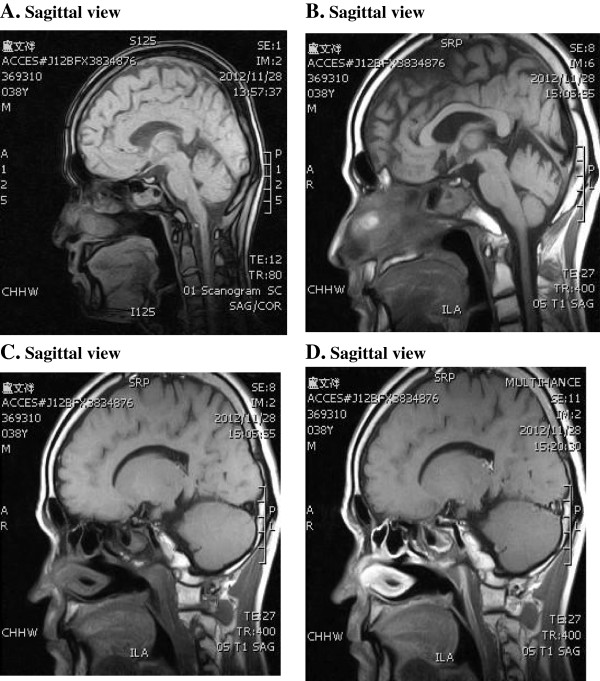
MRI scanogram of patient Lu’s brain. The sagittal views (pictures A-D) reveal the hypophysis has been injured severely and the nasal pharyngeal area is severely deformed.
